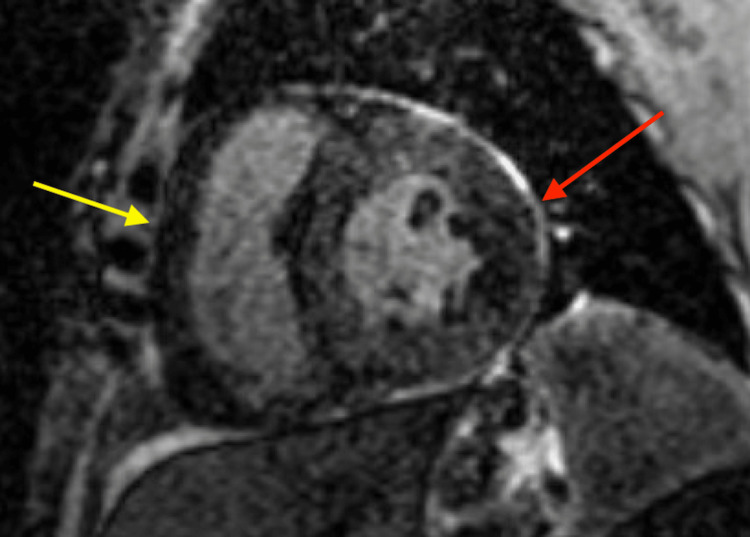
Cardiac magnetic resonance image with delayed gadolinium enhancement, displaying a thickened right ventricular myocardium (yellow arrow) and transmural thickening of the left ventricular myocardium (red arrow)
